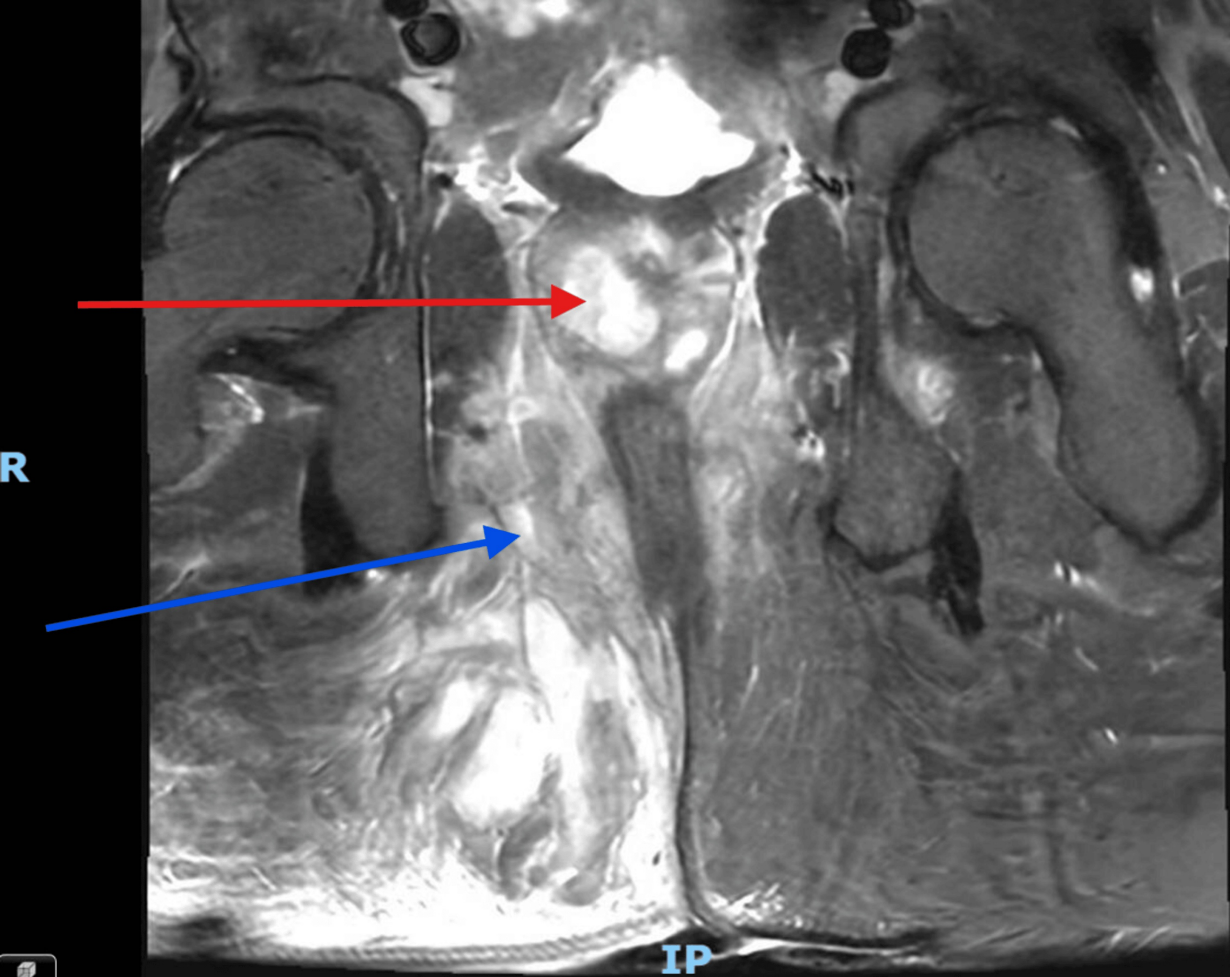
MRI of the prostate taken five days after the initial presentation to the hospital. The red arrow points to the prostate abscess within the prostate gland. The blue arrow points to surrounding tissue edema, including inflammation of the right ischiorectal fossa and gluteus maximus. The letter R depicts the right-hand side of the patient, and IP depicts the inferior-posterior of the patient.
